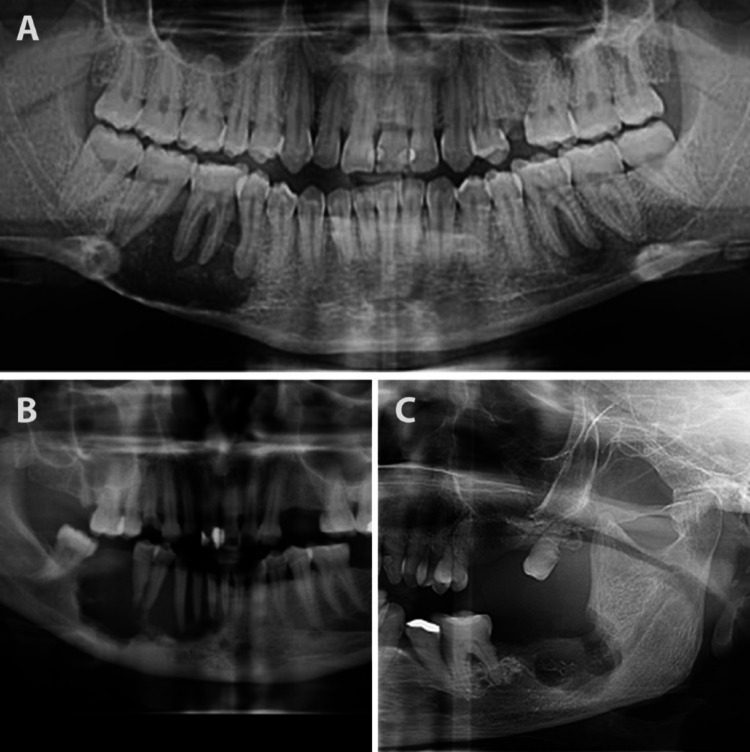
Radiographic features of malignant odontogenic tumors (MOTs) a Ghost cell odontogenic carcinoma. Unilocular lesion in the mandibular molar region (case 05) b Ameloblastic carcinoma. Unilocular radiolucent lesion involving both the anterior and posterior regions of the mandible (case 20). c Primary intraosseous carcinoma, NOS. Multilocular radiolucent lesion with scalloped margins located in the posterior mandibular region (case 02)
